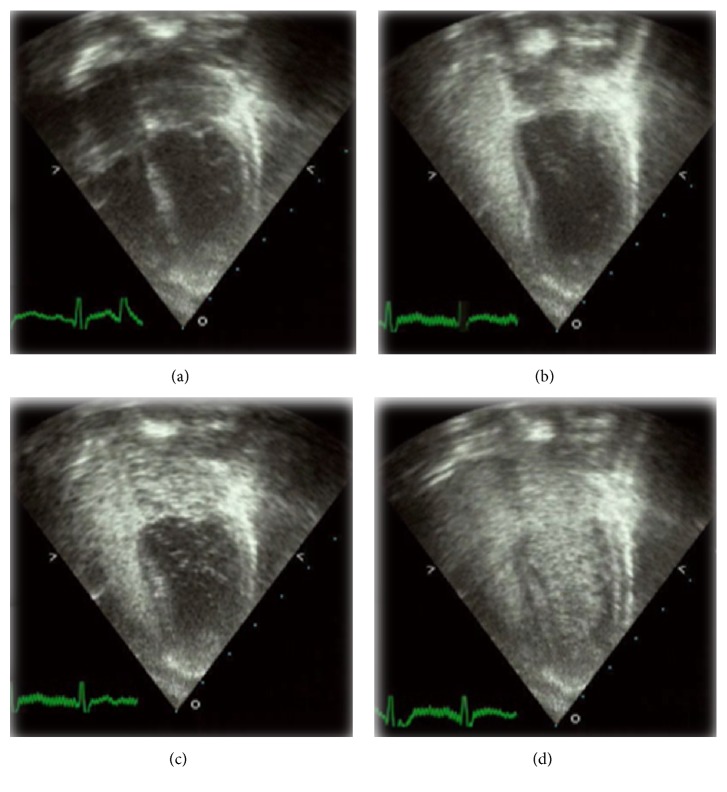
Chest wall echocardiography using agitated saline particles in patient 1 showing echocardiographic still image depicting all four chambers before instillation of agitated saline (a), agitated saline filling the right side of the heart (b), and the left side after a few heart beats (c, d).
